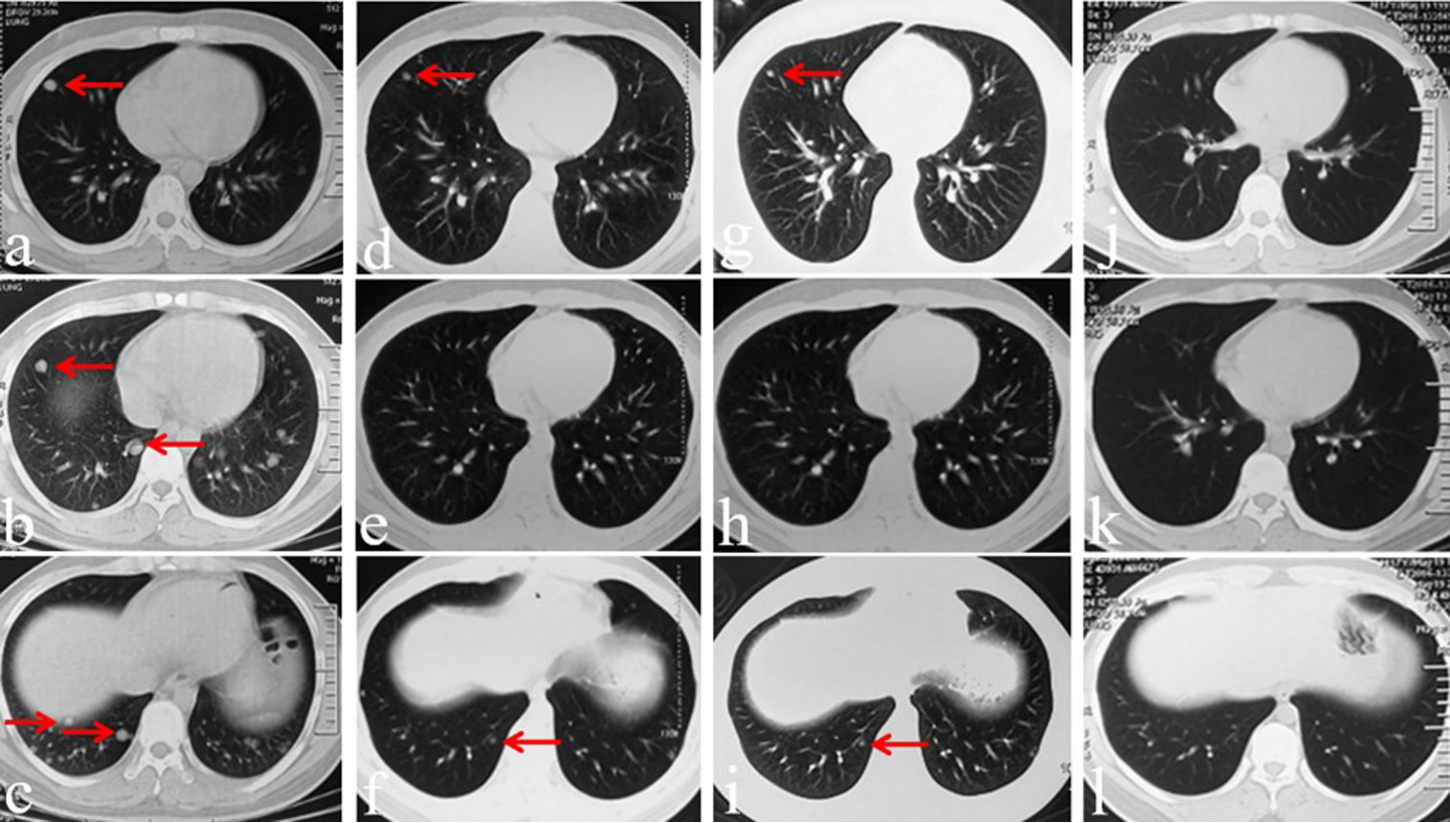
CT images before, during, and after apatinib therapy May 2015 CT images collected before apatinib therapy (a-c). June 2015 CT images collected one month after apatinib therapy, showing response to treatment presenting as secreased lesion numbers and sizes (d-f). September 2015 CT images collected three months after apatinib therapy, showing the disappearance of nearly all of the metastatic leasions (g-i). June 2016 CT images collected three months after apatinib therapy was stopped (j-l). No disease relapse and no new nodules were observed. No disease relapse and no new nodules were observed.
